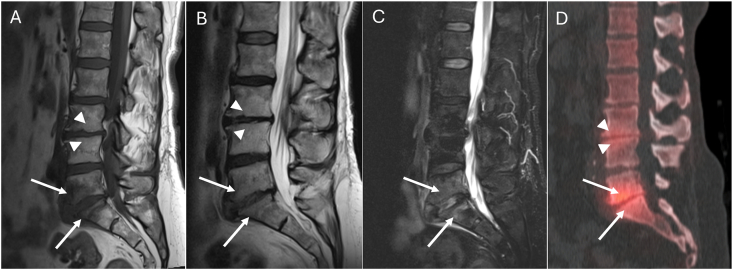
Example of concordance between MRI and SPECT-CT. Sagittal T1-weighted (A), sagittal T2-weighted (B) and STIR sequence (C) images demonstrate Modic type 1 changes at L5-S1 (arrows) and Modic type 2 changes at L3-L4 (arrowheads), with associated reactive changes on sagittal reformatted bone SPECT-CT (D) fused image. At L5-S1, Modic type 1 changes are indicated by hypointense signal in the vertebral bodies adjacent to the L5-S1 disc space on the T1-weighted image, with hyperintense signal on the T2-weighted sequence and STIR image consistent with marrow edema, and there is associated increased radiotracer uptake compared to the background signal in the rest of the vertebrae. At L3-L4, Modic type 2 changes are indicated by hyperintense signal in the vertebral bodies adjacent to the L3-L4 disc space on the T1-weighted image, hyperintense signal on the T2-weighted image consistent with fatty marrow change compared to the background signal in the rest of the vertebrae, and there is associated increased radiotracer uptake as well.
